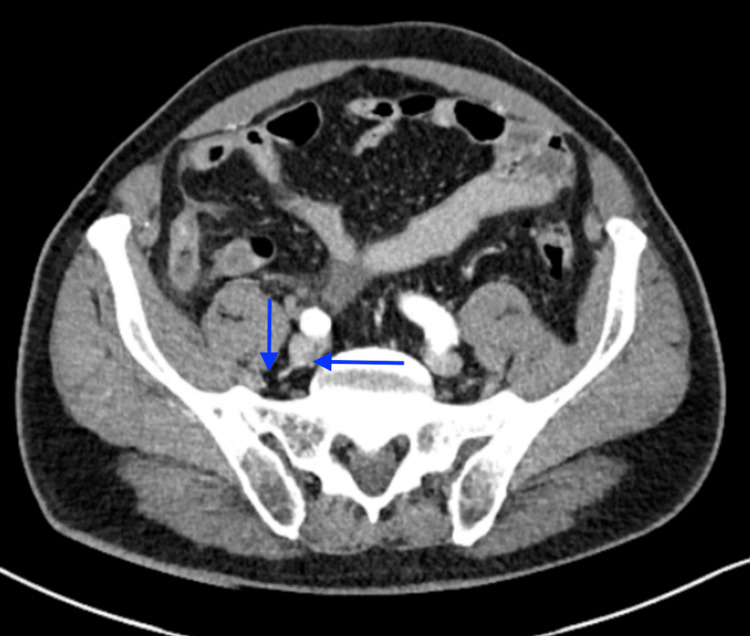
CT scan of the abdomen and pelvis performed six months earlier during the patient’s presentation with acute perforated appendicitis, demonstrating two calcified appendicoliths in the right lower quadrant, near the inflamed and perforated appendix.
